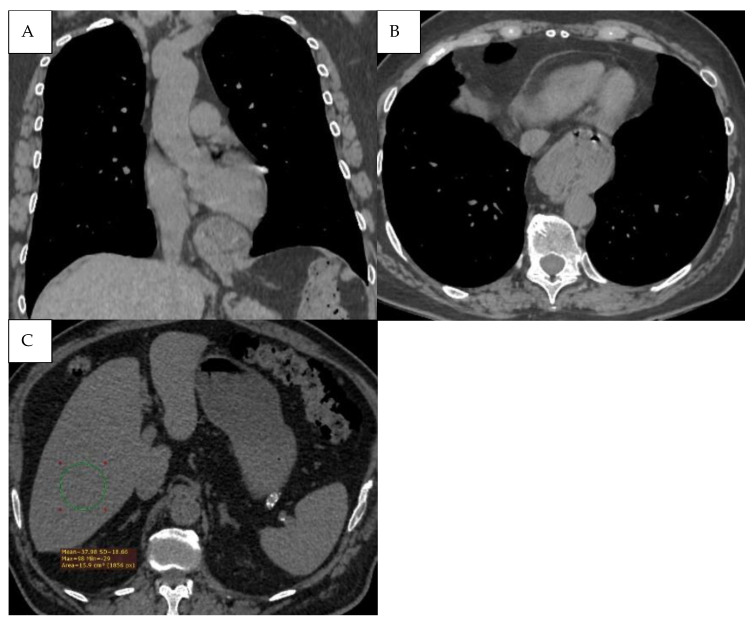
(A,B) CT images shows a hiatal hernia (type 1), with a part of the stomach protruding through the esophageal hiatus into the thoracic cavity. (C) Abdominal CT image showing hepatic steatosis with diffusely decreased liver attenuation (37.98 HU). The green circle indicated the region of interest for liver attenuation measurement.
